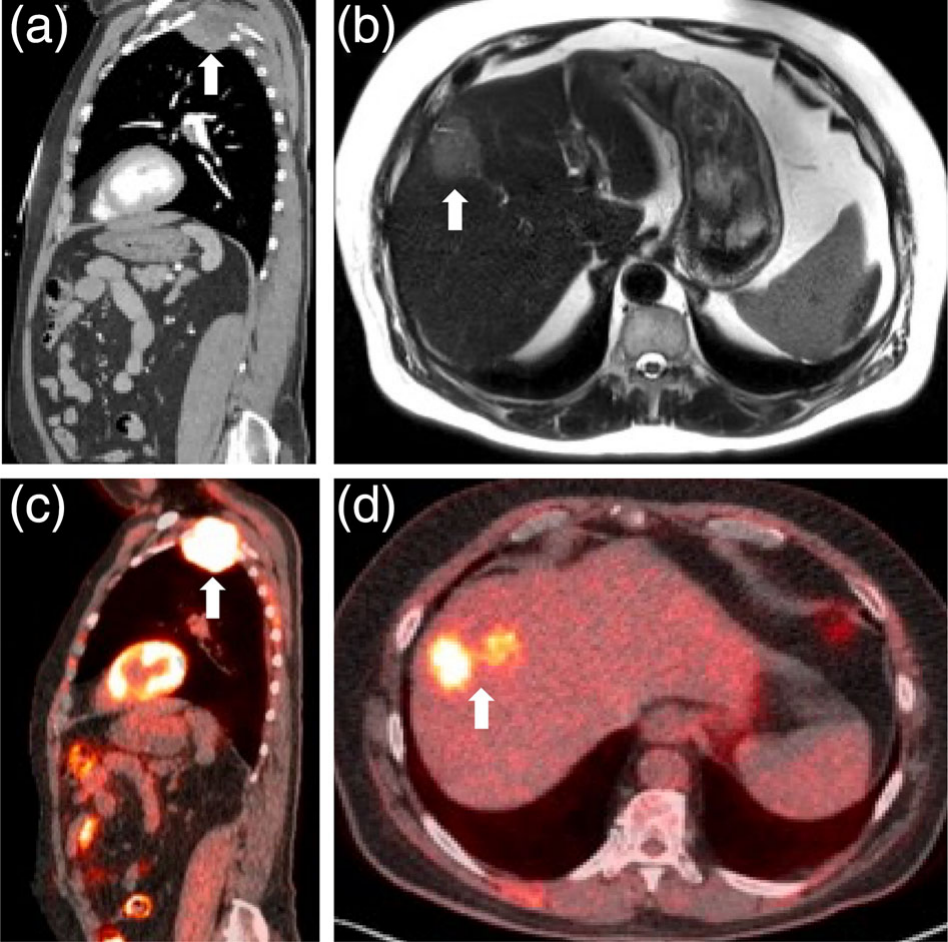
Imaging. (a) Initial sagittal computed tomography (CT)‐angiogram demonstrating a superior sulcus tumor (arrow). (b) T2W axial image demonstrating a mildly hyperintense mass (arrow) in the right hepatic lobe. (c) Sagittal staging positron emission tomography (PET)‐CT image confirming an F‐fluorodeoxyglucose (FDG)‐avid left apical mass (arrow) with interval growth. (d) Axial staging PET‐CT image confirming a lobulated heterogeneously FDG‐avid mass (arrow) in the anterolateral right hepatic lobe.
